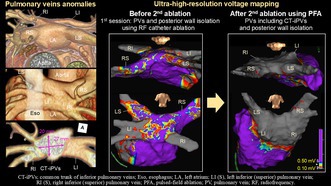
A 76‐year‐old man was referred to our hospital for the recurrence of paroxysmal atrial fibrillation (AF). Two years earlier, the patient had undergone radiofrequency catheter ablation for a left atrial (LA) posterior wall isolation in addition to pulmonary vein (PV) isolation at another hospital. Three‐dimensional reconstruction computed tomography demonstrated a common trunk of the inferior PVs (CT‐iPVs; Figure 1). Ultra‐high‐resolution voltage mapping during coronary sinus pacing with an Advisor™ HD Grid mapping catheter, Sensor Enabled™ (Abbott, Minneapolis, MN, USA) revealed recurrent conduction in the left and right superior PVs. The distal portions of both inferior PVs were electrically isolated, but the conduction resumed in the CT‐iPVs (Figure 2A–D; Movie S1). Furthermore, conduction of the LA posterior wall was also noted, with a little evidence of ablation of the LA roof and lower part of the LA posterior wall (Figure 2A–D). Because the anterior–posterior diameter of the left atrium was extremely small (30 mm) and the CT‐iPV ostium was not round (long/short diameter, 27/20 mm; Figure 1D), catheter manipulation and stabilization in the LA and balloon occlusion at the CT‐iPVs ostium were expected to be difficult. The CT‐iPVs protruded posteriorly, and the left inferior PV was proximal to the esophagus (Figure 1B,C). Therefore, pulsed‐field ablation (PFA), which allows precise catheter placement at the target site along a guidewire and does not require a complete occlusion of the PV for ablation, was performed using a circular catheter (PulseSelect™, Medtronic, Minneapolis, MN, USA). For the isolation of the CT‐iPVs, a guidewire was inserted into the distal portion of each inferior PV, and the PFA was delivered at the ostium of the CT‐iPVs. With 31 PFA applications delivered to the conduction resumption sites, the LA posterior wall, including the PVs, was completely electrically isolated (Figure 2E–H). No untoward events or recurrences were recorded during an 8‐month follow‐up without any antiarrhythmic drugs.
